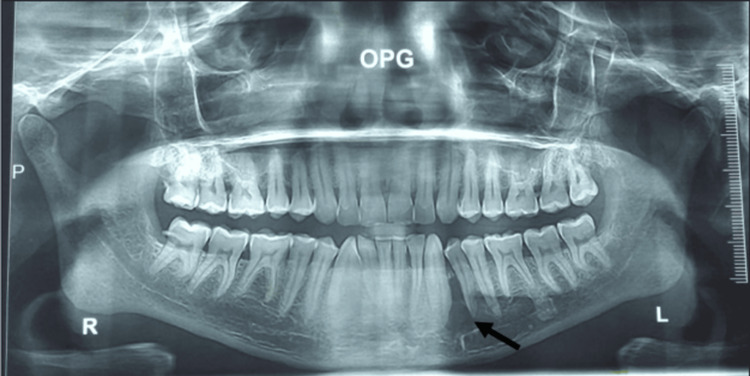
OPG depicted a well-defined, unilocular, completely radiolucent lesion with corticated margins presenting between 33 and 34 involving the periapical area OPG: orthopantomogram
